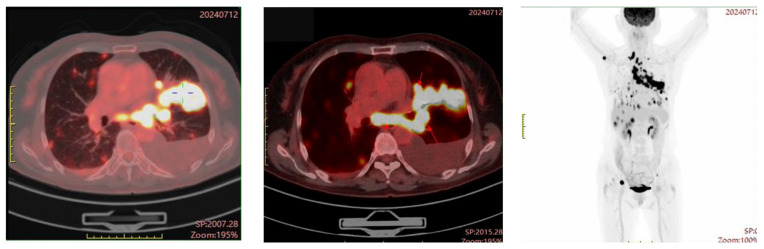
PET-CT at diagnosis. The red arrows indicate the hypermetabolic primary tumor in the left lung and the metastatic lesions.
